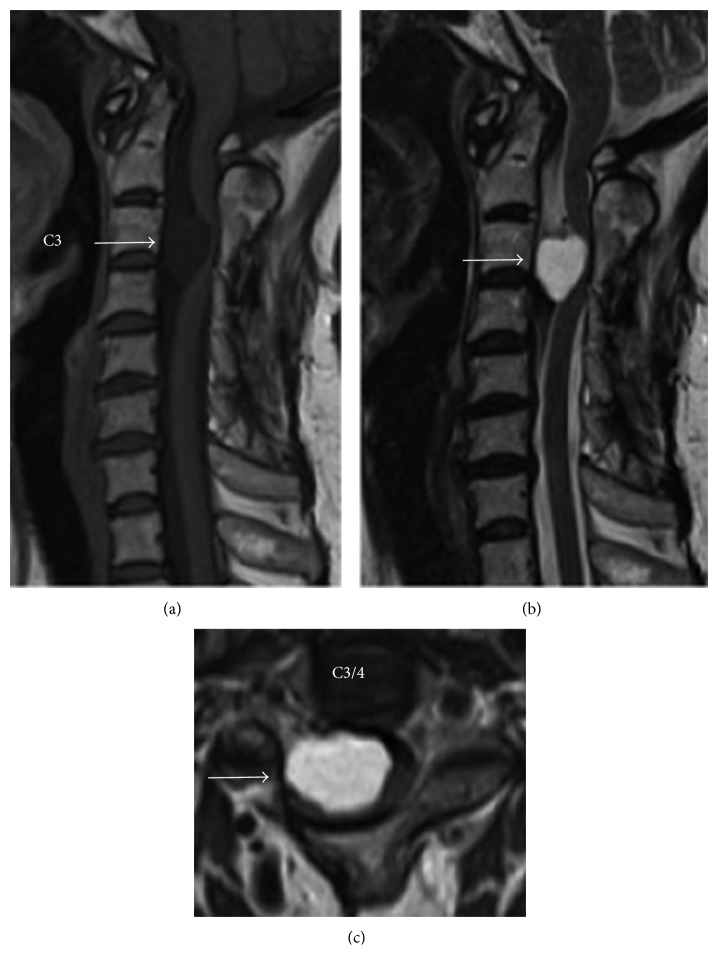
Magnetic resonance imaging (MRI) showing the intradural extramedullary cystic mass lesion. The spinal cord was completely stretched out over this ventral cyst (arrows). (a) T1-weighted sagittal image. (b) T2-weighted sagittal image. (c) T2-weighted axial image.
